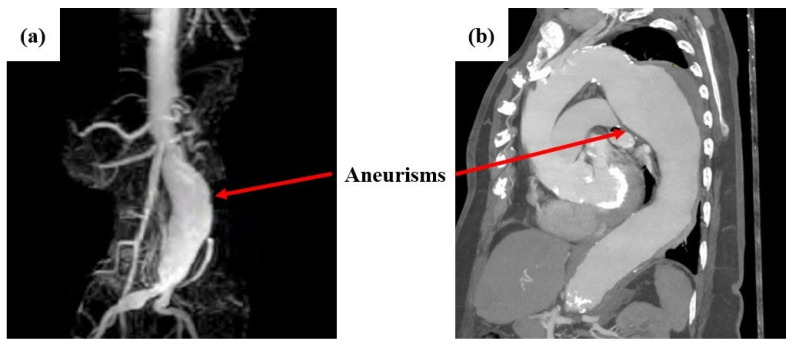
Indicative images of abdominal aortic aneurysms captured with: (a) MRA and (b) MRI imaging [9,10].
